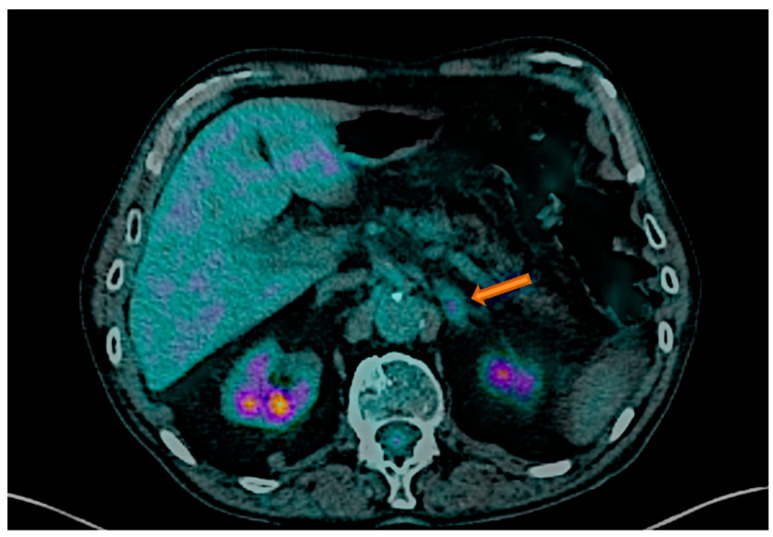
[^18^F]FDG PET/CT of 59-year-old female with history of breast cancer. Left low-density adrenal secreting-lesion indicated by the arrow (SUV max: 2.59; UH −10.65).
